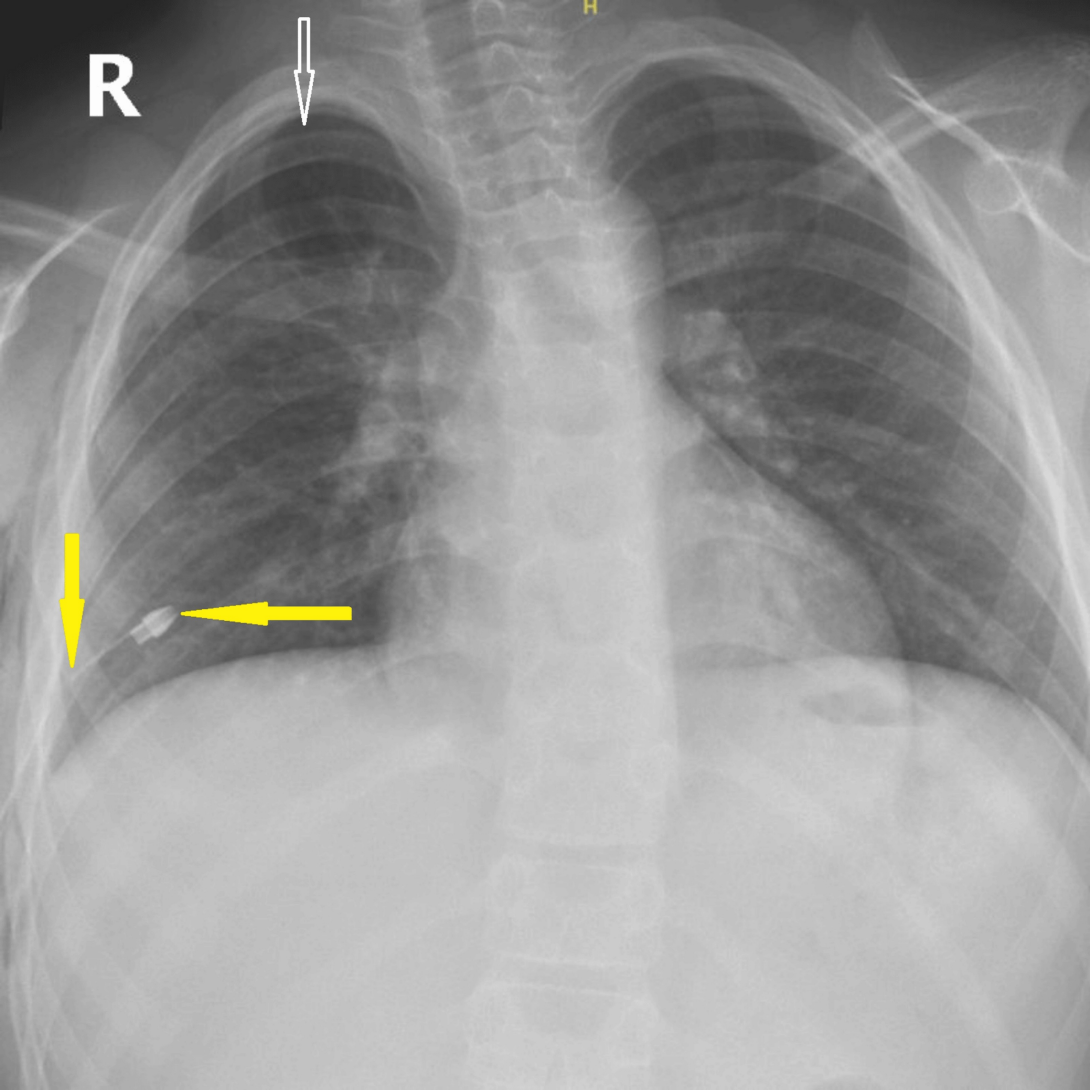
Post-drainage chest radiograph Chest X-ray showing re-expansion of the right lung following chest tube insertion. Solid yellow arrows indicate the chest tube position, and the hollow white arrow indicates a small residual apical pneumothorax pocket, which resolved later during follow-up. The tube side holes were confirmed to be within the thoracic cavity on subsequent CT imaging.
